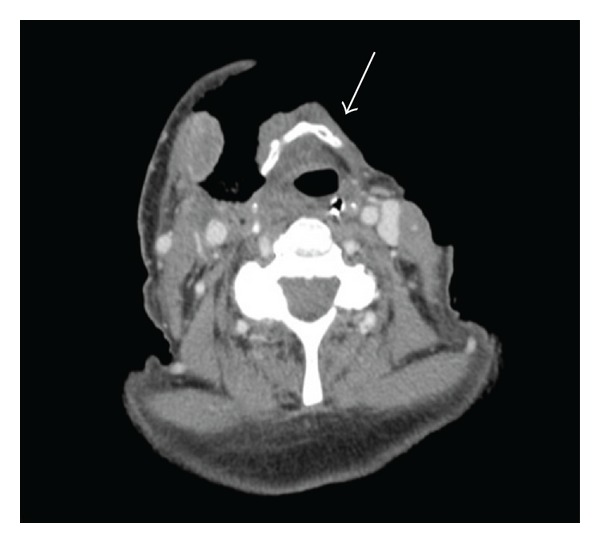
Postoperative CT scan at level of the hyoid. Note: the extensive loss of soft tissue in the anterior neck compartment (arrow).
